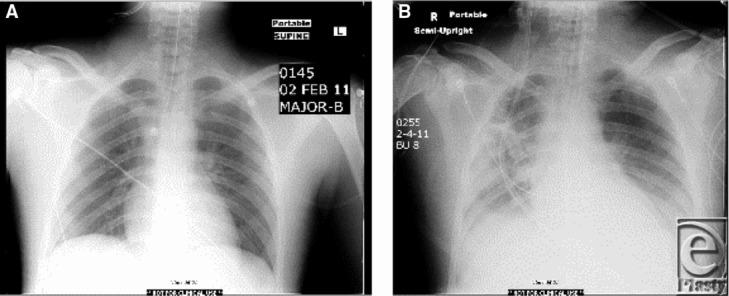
Cody's normal chest xray on admission. 7b. Pulmonary edema day 2.
